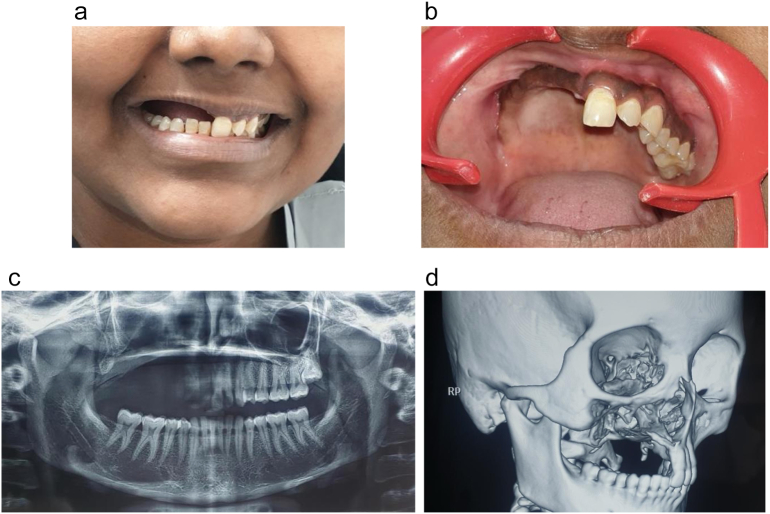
The patient's clinical presentation. A. The extraoral view of the patient. B. The intraoral view displays the patient's right partial maxillectomy following soft tissue approximation and healing. C. A panoramic radiograph revealing the right maxillectomy with complete mandibular and left-side dentition. D. A 3D Cone beam CT showing the maxillary defect.
